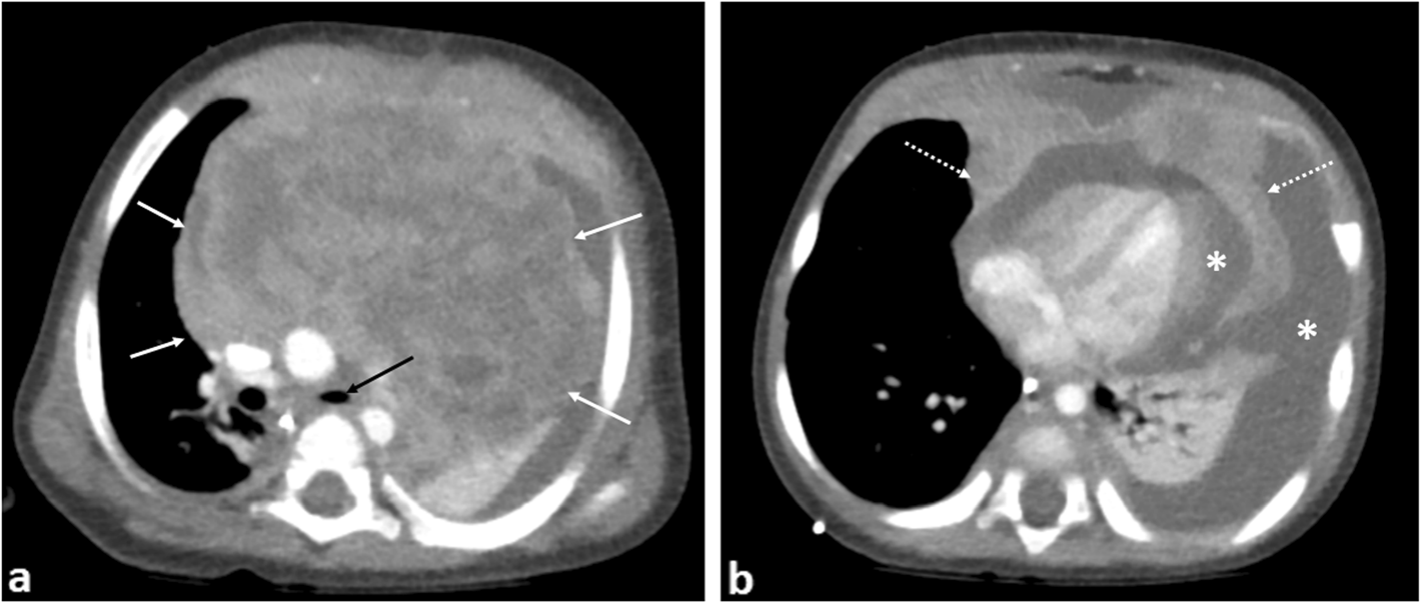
An 8-month-old boy with T-cell lymphoblastic lymphoma. a Contrast-enhanced axial CT image of the superior mediastinum and (b) at the level of the cardiac ventricles demonstrate a large heterogeneously enhancing anterior mediastinal mass (white arrows) with pericardial invasion (dashed arrows). There is narrowing of the left main bronchus (black arrow), left lung collapse and a large pericardial and left pleural effusion (asterisks)
